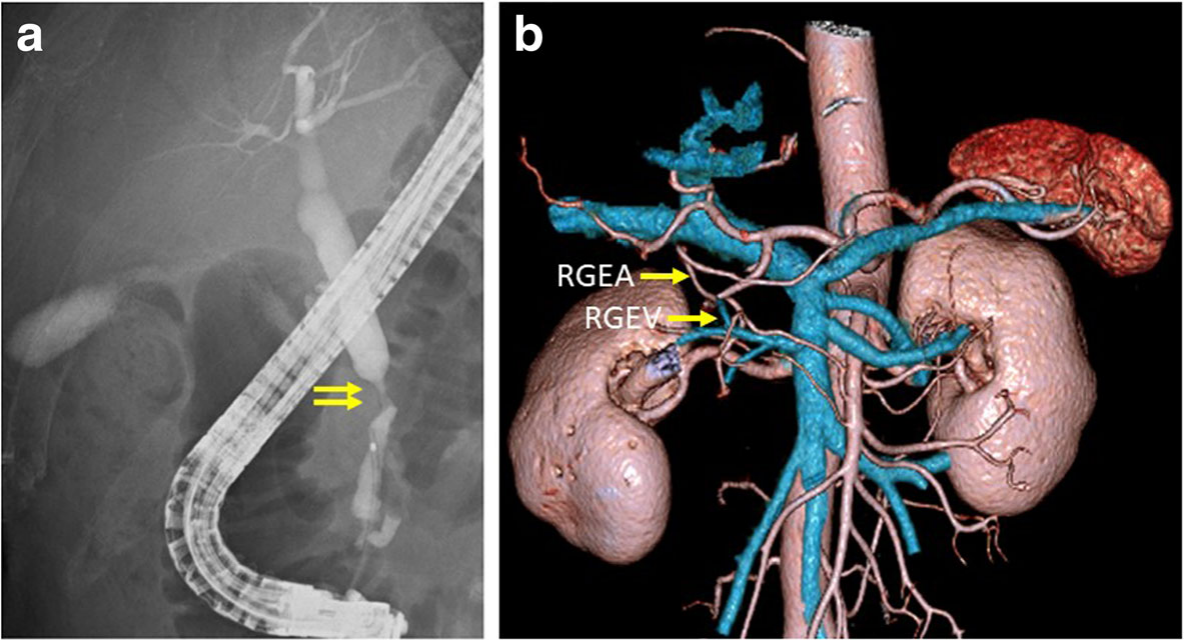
a ERC showed stenosis of the common bile duct with a diameter of 15 mm. b CT reconstructing blood vessels
